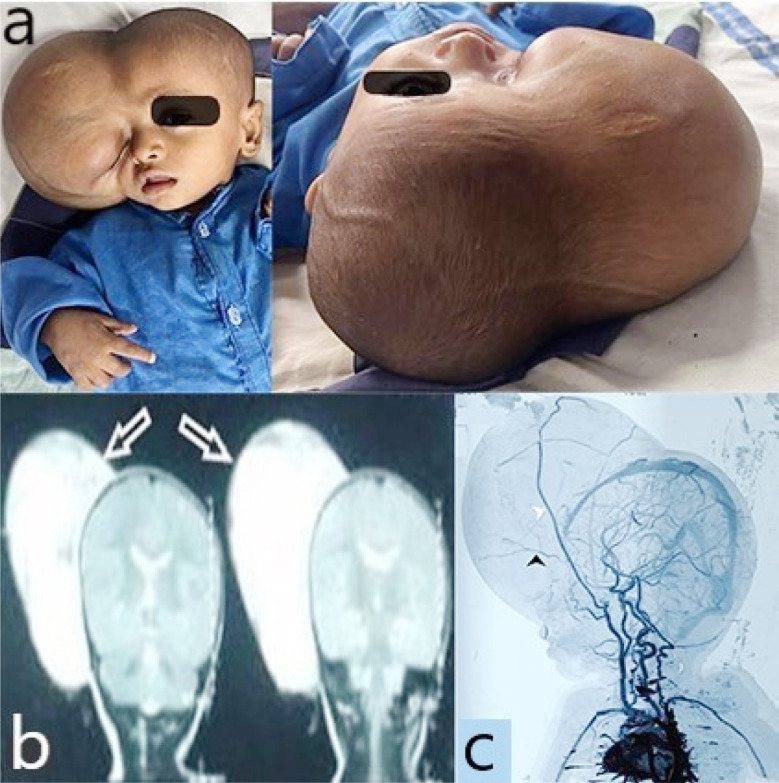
(a) Pictures taken before the surgery, depicting a massive scalp mass. (b) Coronal T2W images showing a large hyperintense fatty lesion (black arrows). (c) CTA brain sagittal image showing the arterial supply of the lesion from superficial temporal artery (white arrowhead) and ophthalmic artery (black arrowhead).
